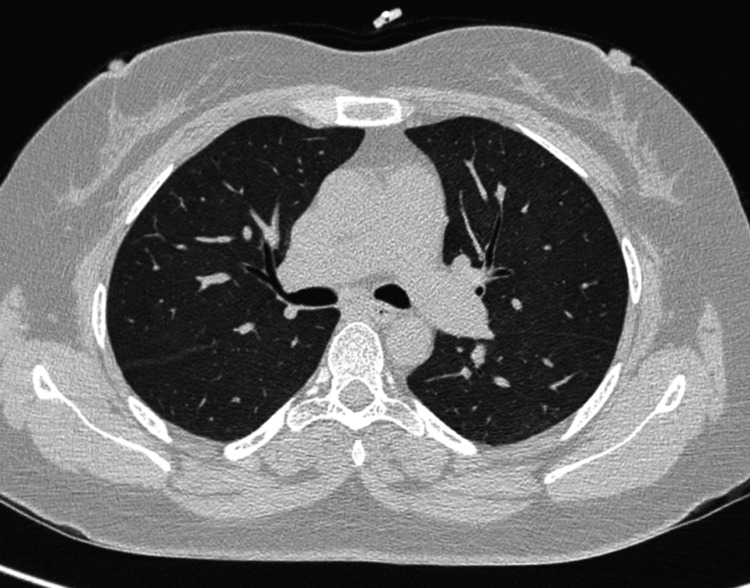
High-resolution CT of the thorax showing no pleuro-parenchymal abnormality.
